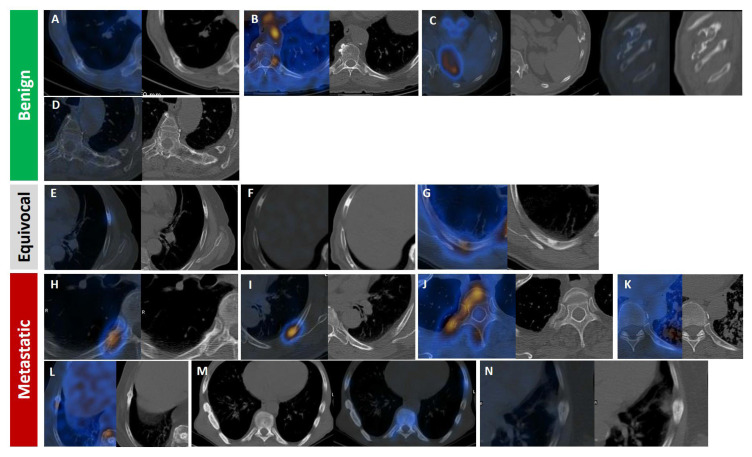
Radiologic appearance of PSMA-avid rib lesions in prostate cancer patients, categorized by likelihood of malignancy: benign (A–D), equivocal (E–G; all isolated), and malignant (H–N). Fracture line with callus formation and faint PSMA uptake (A). Focal moderate uptake at the costovertebral joint, consistent with degenerative changes (B). Well-defined expansile non-PSMA-avid lytic lesion at the costochondral junction, likely a benign chondroid lesion (C). Left transverse process connected to an adjacent isolated rib (D). Focal lytic–sclerotic lesion anterolaterally with moderate uptake. (F) Focal dense sclerosis without uptake (E). Eccentric/mural focal sclerosis with moderate uptake (G). Focal intense uptake with periosteal reaction and sclerosis at the costovertebral rib portion (H). Focal intense uptake in the posterior rib arc with ground-glass appearance (I). Focal intense uptake with mild sclerosis in the vertebral body extending to head, neck, and posterior rib arc (J). Focal mild uptake with sclerotic changes in posterior arc amid miliary lung metastasis (K). Linear sclerotic lesion showing moderate PSMA uptake and callus formation, indicating pathologic fracture (L). Linear dense sclerotic changes along lateral rib arc with mild PSMA uptake (M). Periosteal reaction with faint PSMA uptake and adjacent soft tissue involvement (N).
